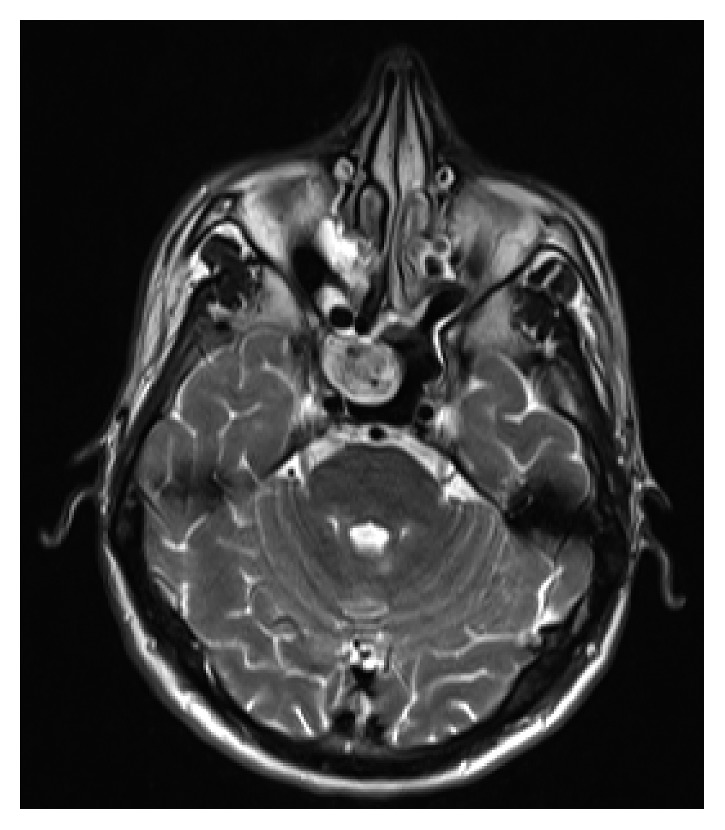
Magnetic resonance image (T-2) of angiofibroma localized in the sphenoid sinus.
